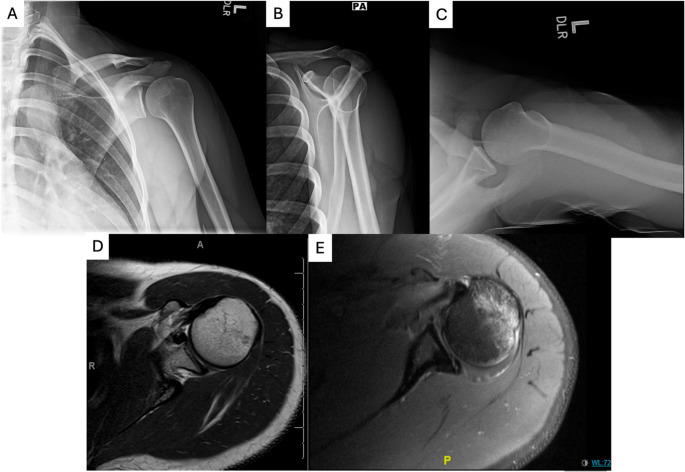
Diagnostic Imaging for Shoulder Instability. (a) AP Oblique View on X-ray to assess glenohumeral alignment and bony defects; (b) Scapular Y View on X-ray to evaluate humeral head positioning and acromion morphology; (c) Axillary Lateral View on X-ray to detect posterior dislocation and glenoid bone loss; (d) Axial View on MRI highlighting anterior labral tear; and (e) Axial View on MRI highlighting a Hill-Sachs lesion
